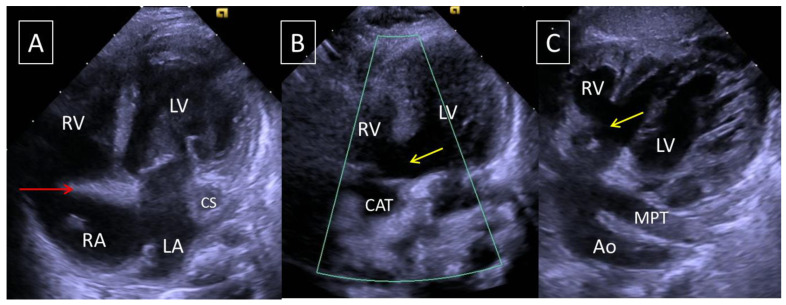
Case 39 (Table 2) with tricuspid atresia and common arterial trunk type 1: echocardiographic views. (A): Four-chamber apical view. Red arrow: absence of right atrioventricular connection (tricuspid atresia). CS, dilated coronary sinus; LA, left atrium; LV, left ventricle; RA, right atrium; RV, right ventricle. (B): Five-chamber apical view. The common arterial trunk overrides the ventricular septum, above a large outlet ventricular septal defect (yellow arrow). CAT, common arterial trunk; LV, left ventricle; RV, right ventricle. (C): Subcostal view. Common arterial trunk type 1. Yellow arrow, outlet VSD; Large outlet VSD. Ao: Aorta; MPT: Main pulmonary trunk.
